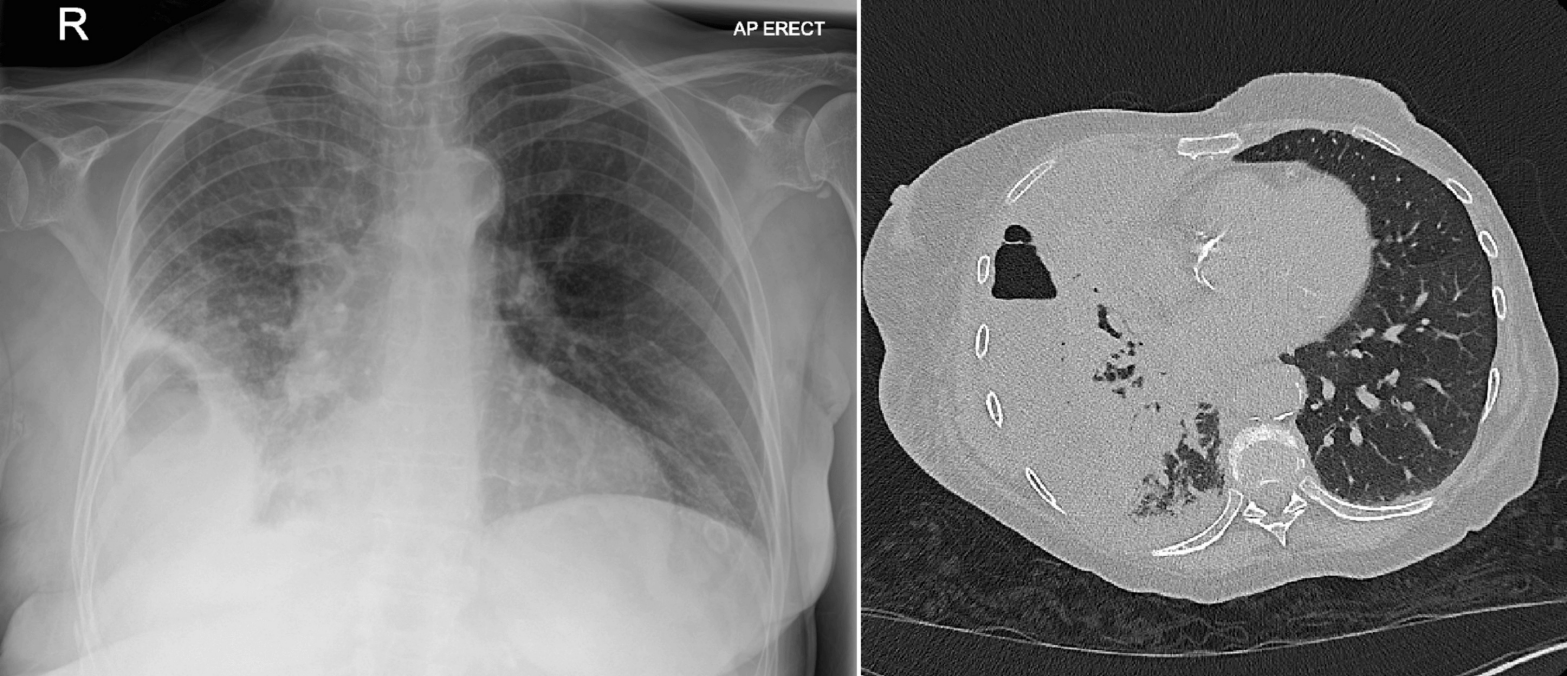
Toward the left side, the chest radiograph at the time of admission. Toward the right side, a cross-sectional image of the CT scan
